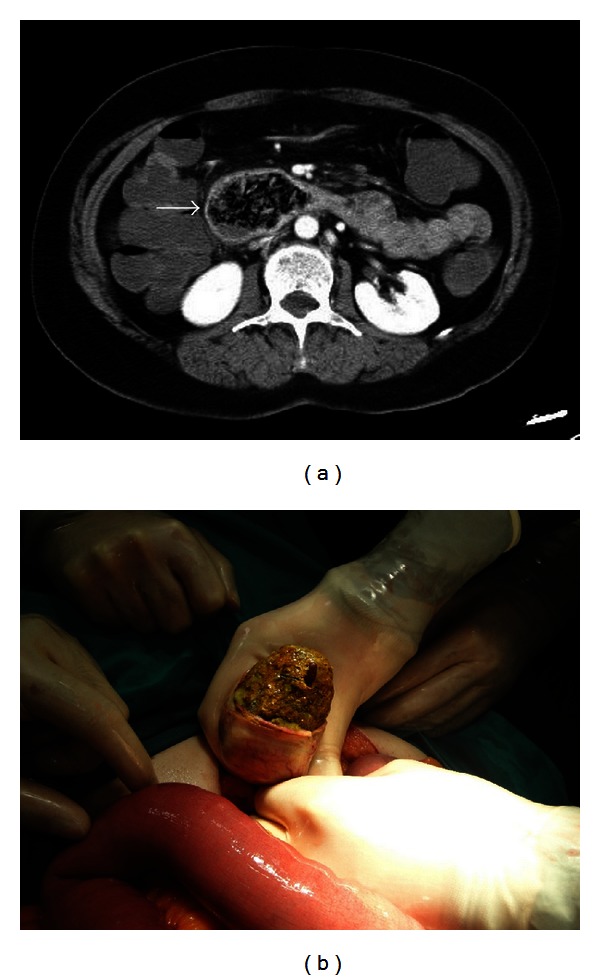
61-year-old female patient. (a) CT findings: an intraluminal ovoid bezoar. Fusiform-shaped seeds were seen in the mass, and wall thickening was seen in the duodenum (arrow). (b) Intraoperative findings: an ovoid-shaped bezoar containing seeds was removed via enterotomy.
